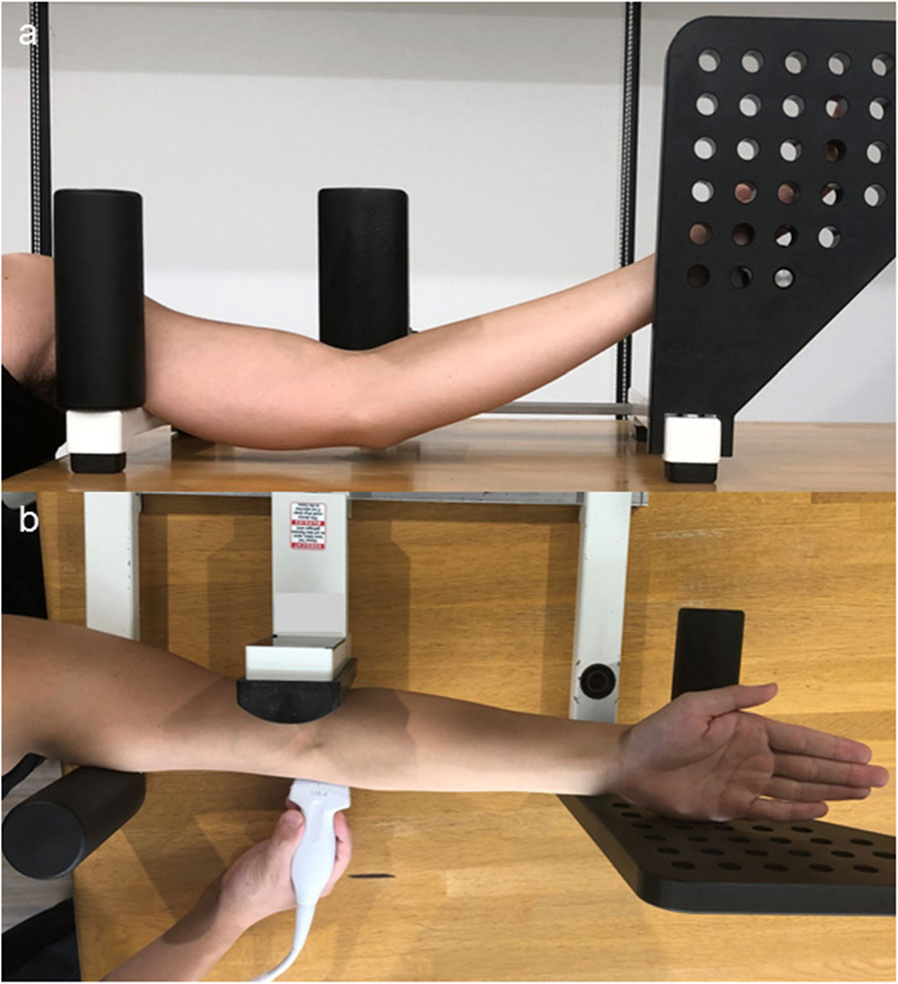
The positioning of the elbow on the Telos stress device. a Subject sitting on a chair with the shoulder at 60° abduction, the elbow at 30° flexion, and the forearm in a supination position. b The ultrasonographic images are obtained from the medial side of the elbow. 50 N of valgus stress is applied to the lateral side of the elbow by the Telos device
